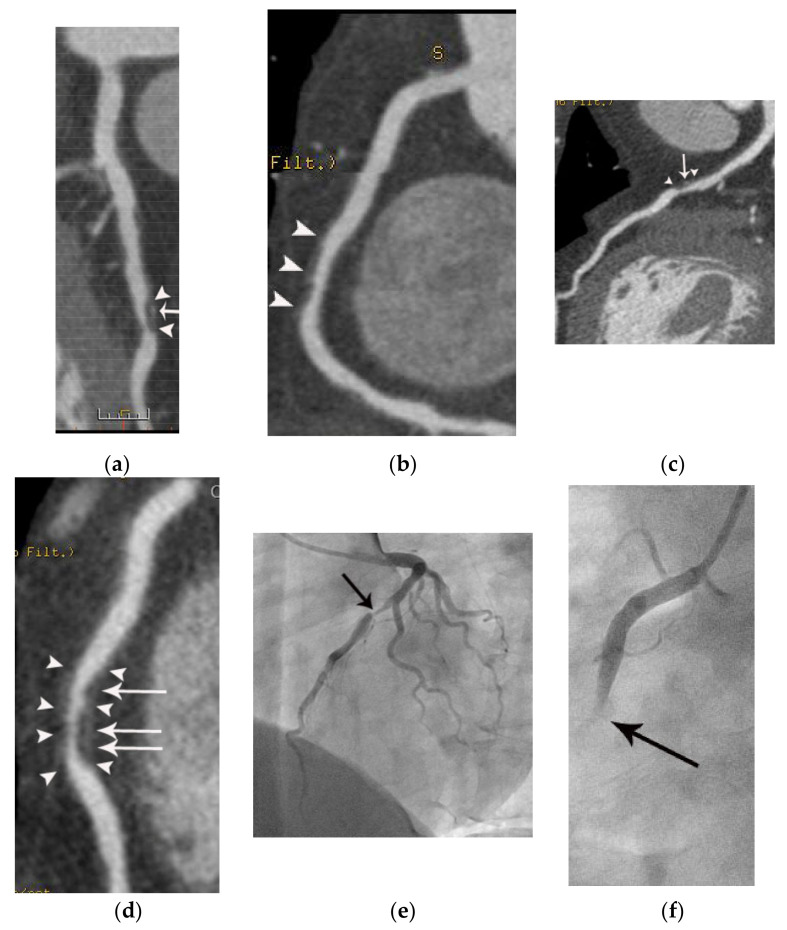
A 46-year-old man presenting with ACS. Coronary CTA was obtained due to multiple cardiac risk factors. Approximately 70% stenoses with mixed plaque (a) and 40–50% stenosis with noncalcified plaque (b) are noted in the mid left anterior descending and mid right coronary artery, respectively. At the 12-month follow-up coronary CTA, the stenosis in the mid left anterior descending coronary artery shows no definite change (c). In contrast, note the progression of plaque (d) in the mid right coronary artery in the degree of coronary stenosis (about a 70% stenosis), the increase in the positive remodeling index (arrowheads in (d)) and the low-attenuation plaque/napkin ring sign (arrows in (d)). Invasive coronary angiography was recommended in this patient, but the patient refused and was lost to follow-up. He presented to the ED with acute chest pain 2 months later. Emergent coronary angiography showed total occlusion indicating a culprit lesion in the same location as the vulnerable plaque in the mid right coronary artery (arrow in (f)). In contrast, the stenosis in the proximal middle left anterior descending coronary artery (nonculprit lesion) showed no interval change (arrow in (e)).
